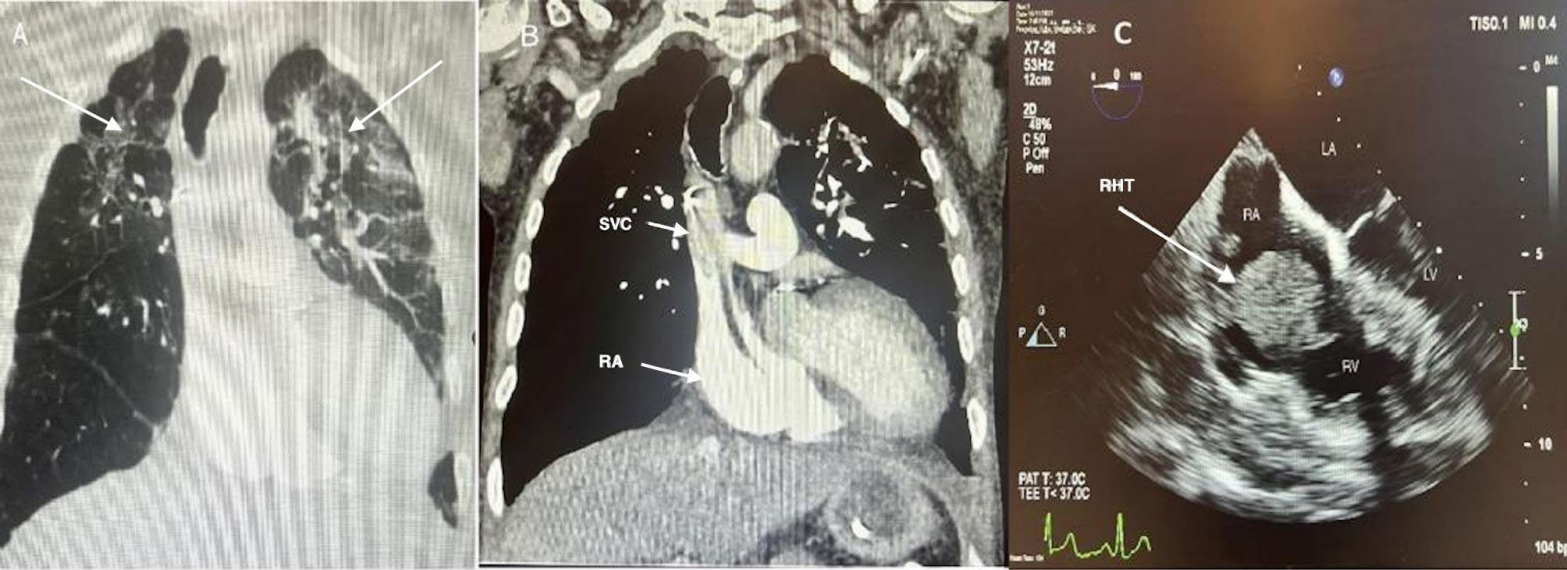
a: CT chest (coronal section) demonstrating bilateral upper and middle zone parenchymal changes; b: CTPA (coronal section) demonstrating thrombus in transit extending into the right atrium (arrows); c: TTE 4 chamber view demonstrating mobile thrombus in the RA prolapsing through the TV
